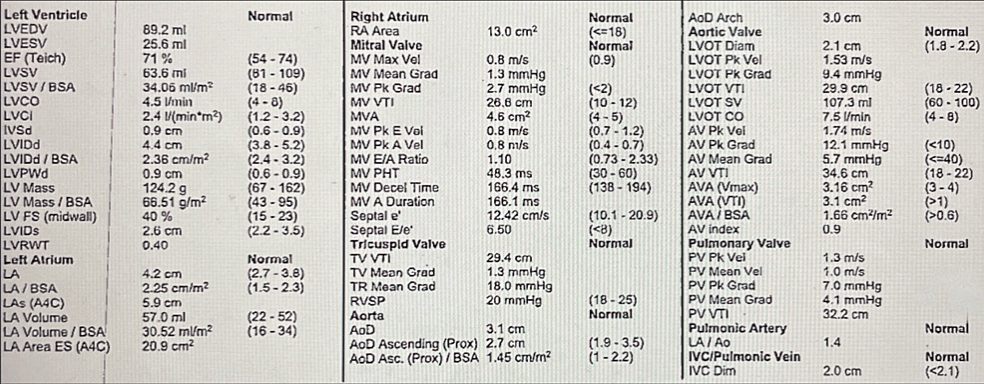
Transthoracic echocardiogram showing no acute abnormalities.
